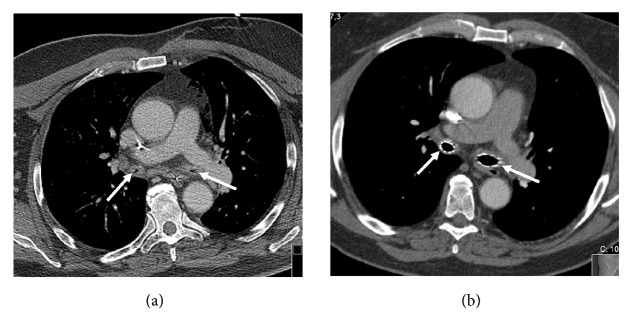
CT imaging before (a) and after (b) stenting. Arrows pointing at main bronchi.
